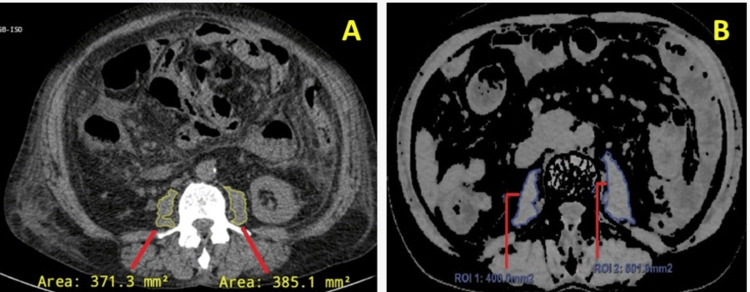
The representative CT images of two cases showing PMA in patients with liver cirrhosis (A) Sarcopenia and (B) non-sarcopenia PMA: psoas muscle area
